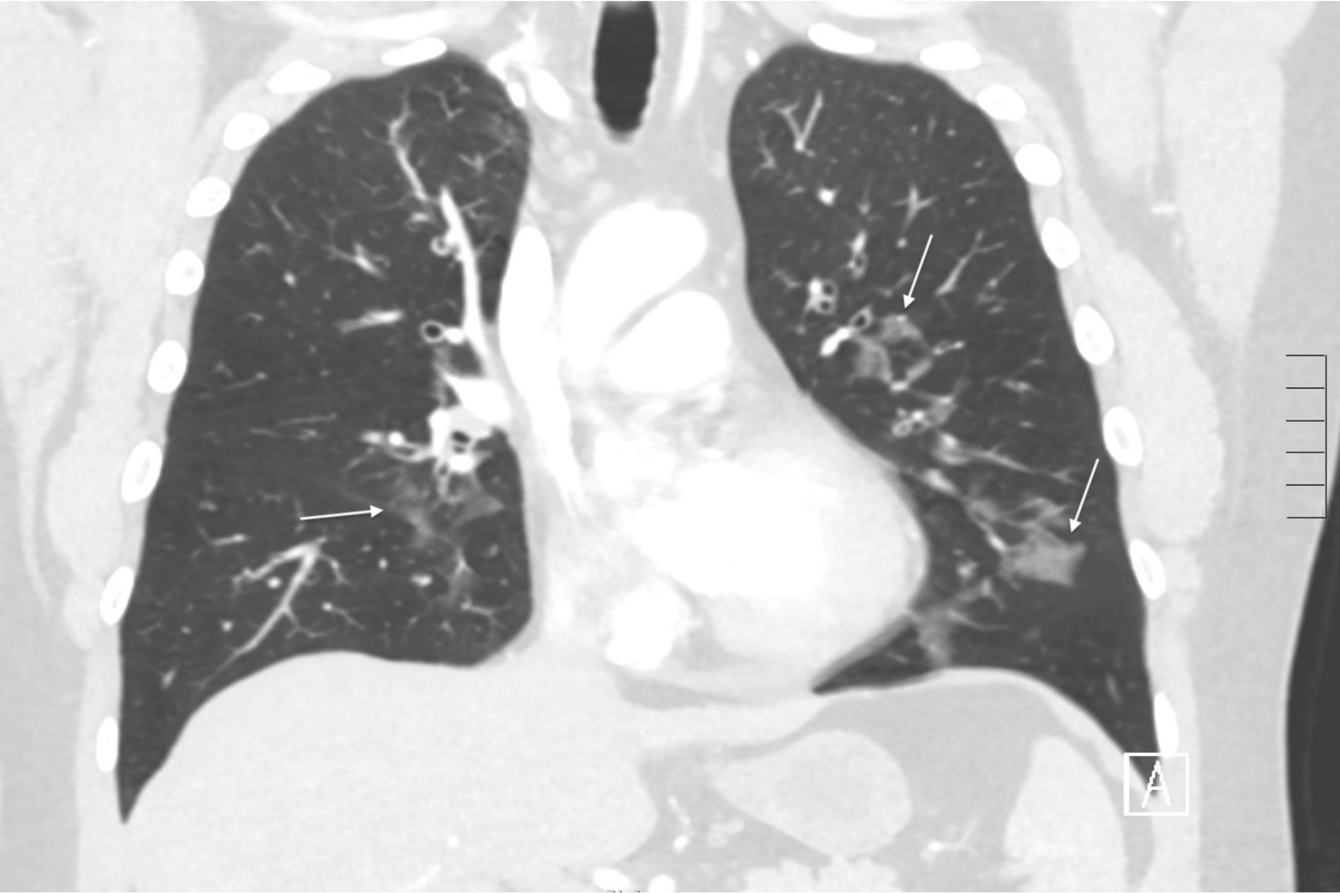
Coronal view of non-contrasted chest CT demonstrating multifocal, predominantly peribronchial ground glass opacities (arrows).
